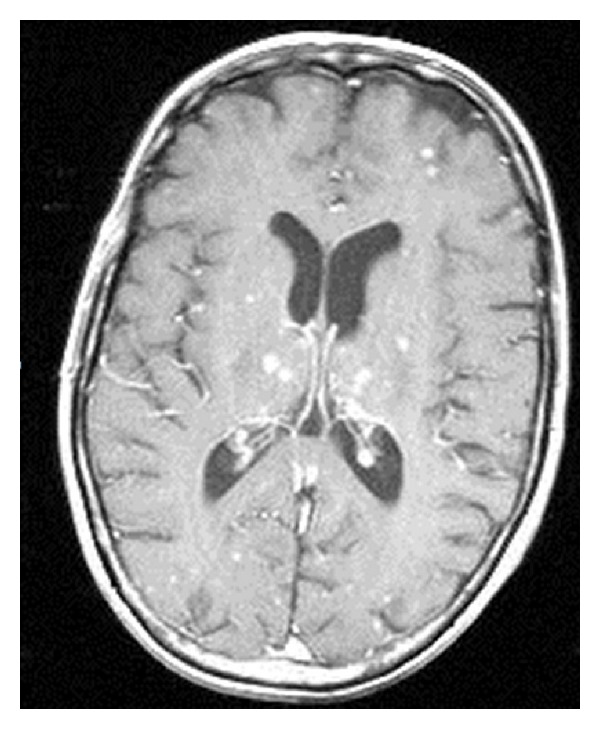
MRI Brain with and without contrast: T1 axial postcontrast image showing diffuse nodule enhancement, most pronounced in the thalamic regions and basal ganglia.
